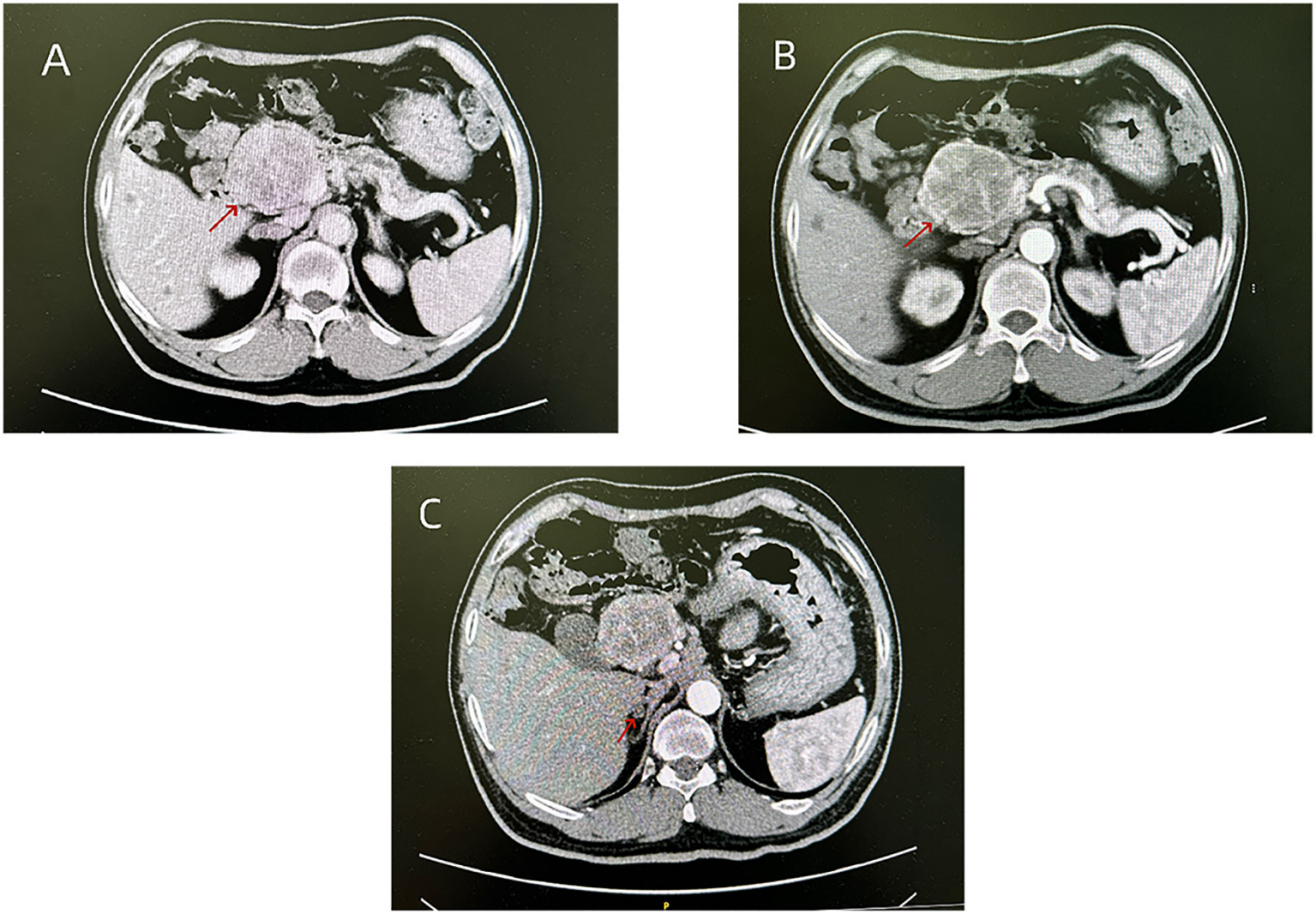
Vascular CT showed a 53*58 mm nodule on the pancreatic head, with uneven enhancement: (A) Venous phase, (B) Arterial phase; CT showed low density nodules in the right adrenal gland (C).
